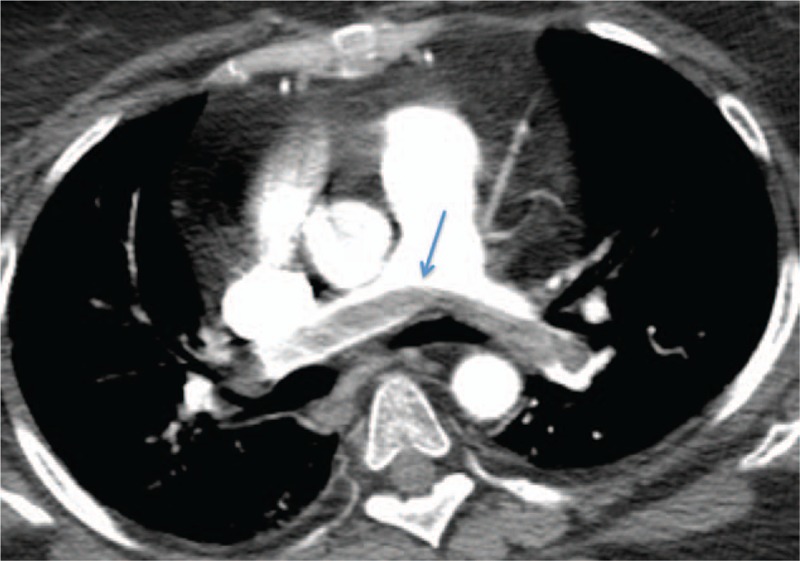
CT angiogram of the chest showing saddle embolus in pulmonary arteries (marked with blue arrow). CT = computed tomography.
